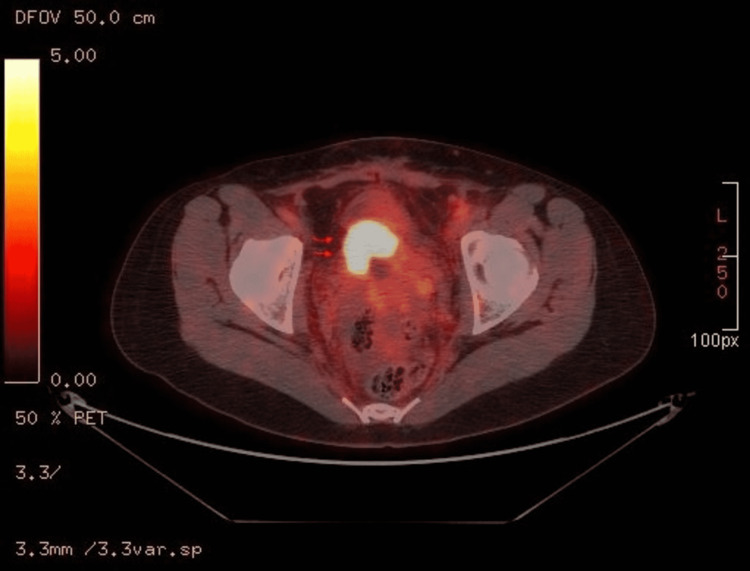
PET-CT showing enlarged intensely FDG-avid cervical mass consistent with cervical carcinoma (SUV of 55.5). PET-CT: positron emission tomography-computed tomography; FDG: fluorodeoxyglucose; SUV: standardized uptake value
